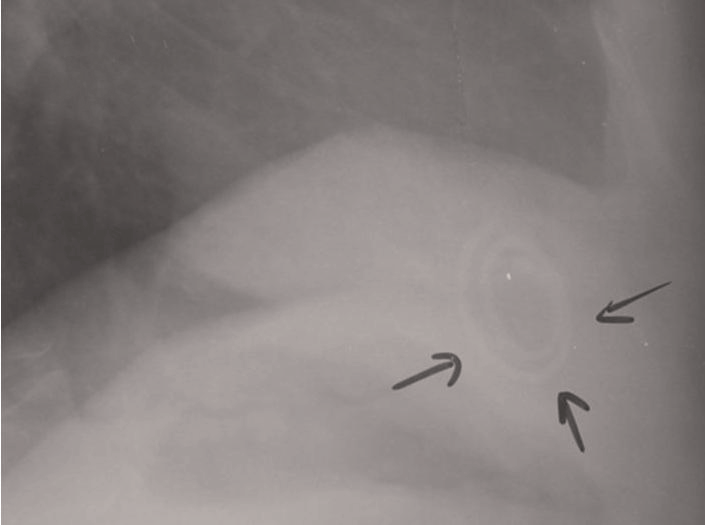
Chest X-ray PA showing the radioopaque IUD in the right subdiaphragmal region.
